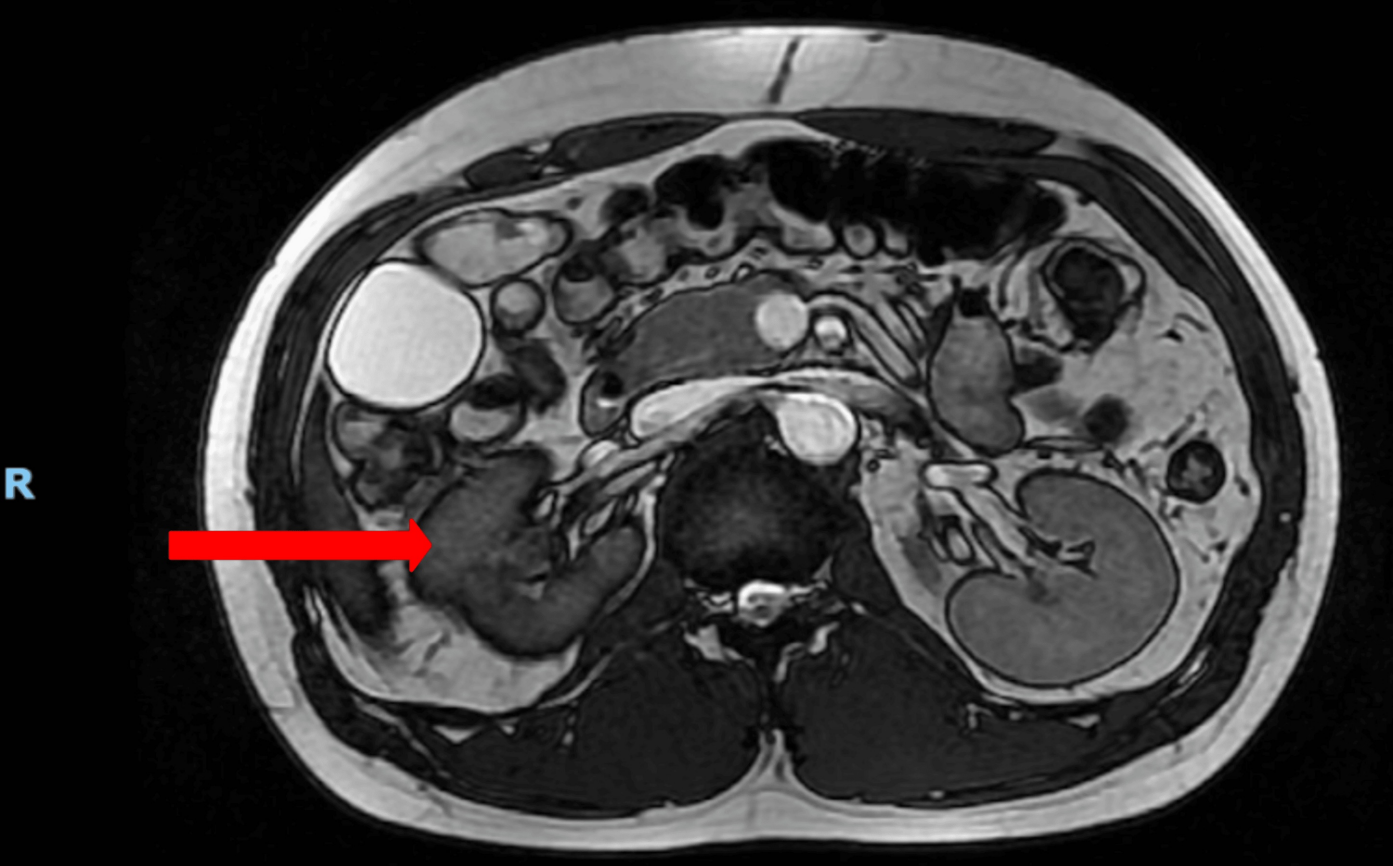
MRI renal (sagittal view) taken two years after initial insult The red arrow points to a resolved perinephric hematoma of the right kidney. The letter R depicts the right-hand-side of the patient.
